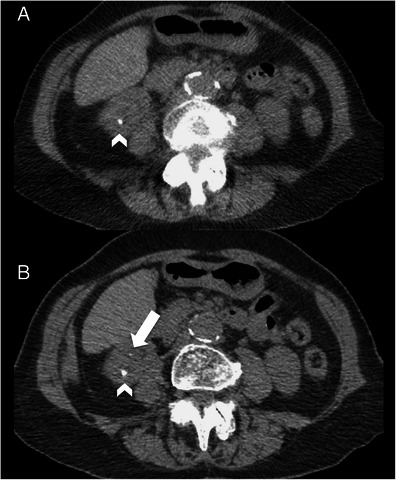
A 53-year-old female patient (BMI = 26.3) with acute left ureteric colic. a Axial low-dose (0.43 mSv) 2-mm non-contrast CT image reconstructed with 70 % ASiR showing a 5-mm calculus in the lower pole of the right kidney (*arrowhead*). b Axial conventional dose (5 mSv) 2-mm non-contrast CT image reconstructed with 40 % ASiR showing an additional 1.7-mm renal calculus in the right lower pole (*arrow*)
